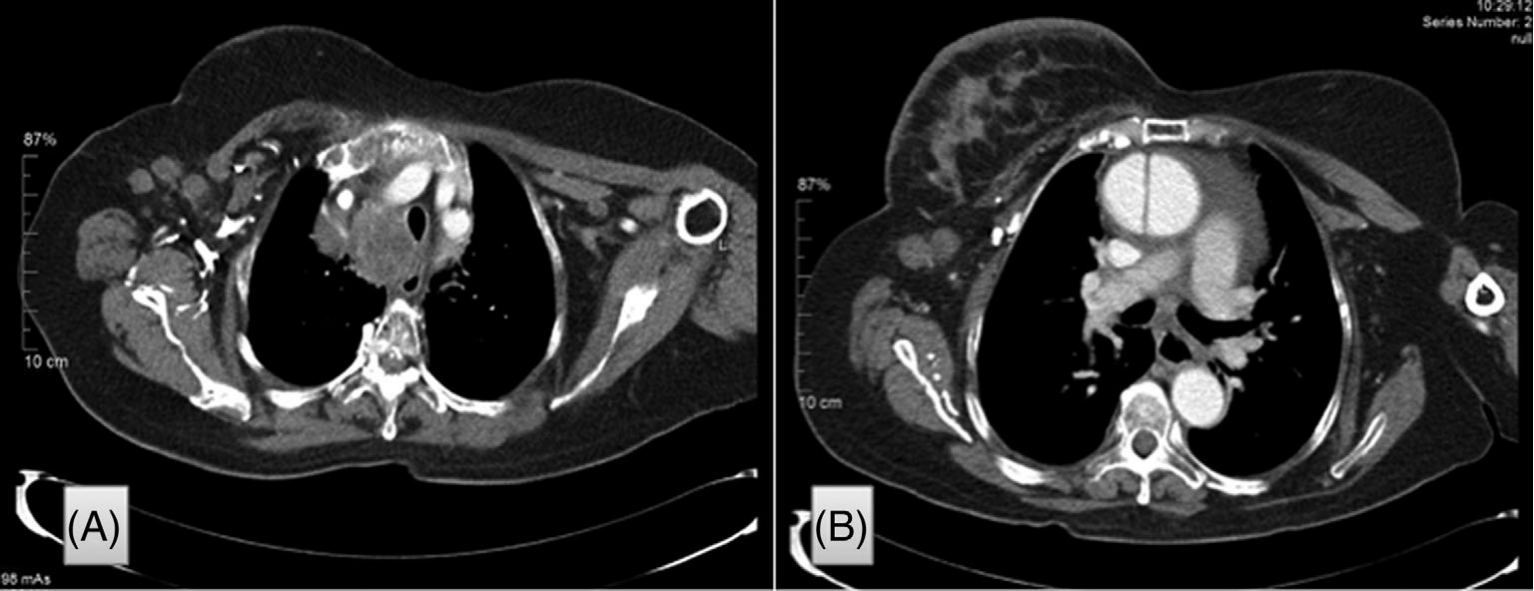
Chest computed tomography scan. A, Enlarged metastatic lymph nodes in the right axillary region. B, Thickened skin on the right breast and enlarged mediastinal lymph nodes
